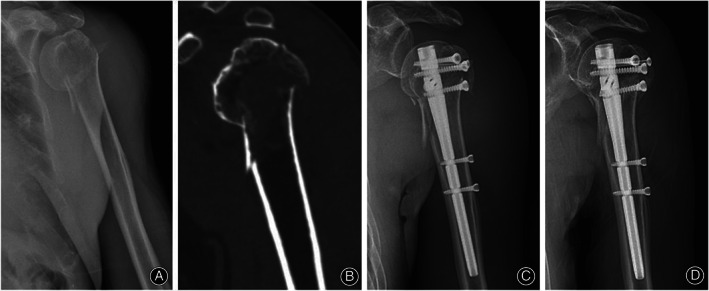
(A) Preoperative radiograph; (B) Preoperative CT scan; (C) Radiograph immediate postoperatively; (D) Radiograph 3 months postoperatively
